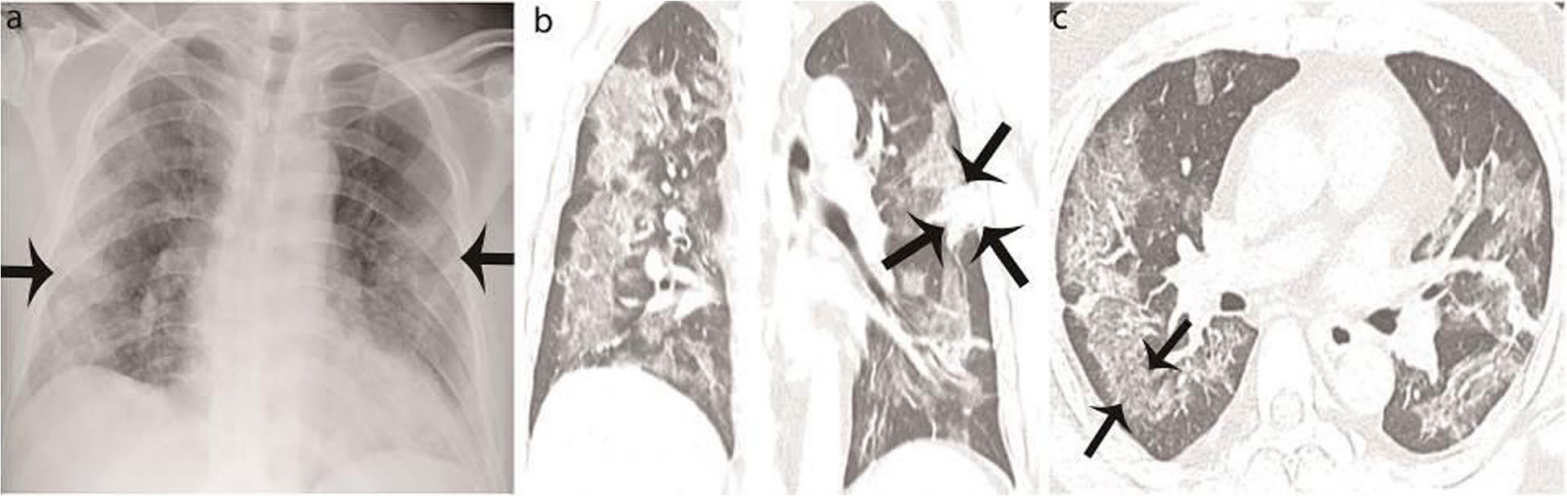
Visualization of the organized pneumonia pattern seen in the disease with X-ray and CT. a. A 52-year-old man. The chest X-ray obtained due to weakness shows peripheral-weighted focal density increases in the bilateral middle and lower zones (arrow). There is an organized pneumonia pattern in the form of crazy-paving accompanied by focal consolidation (arrow), suggesting diffuse alveolar damage and pneumonic infiltration in the coronal b and axial c CT sections of the same patient
